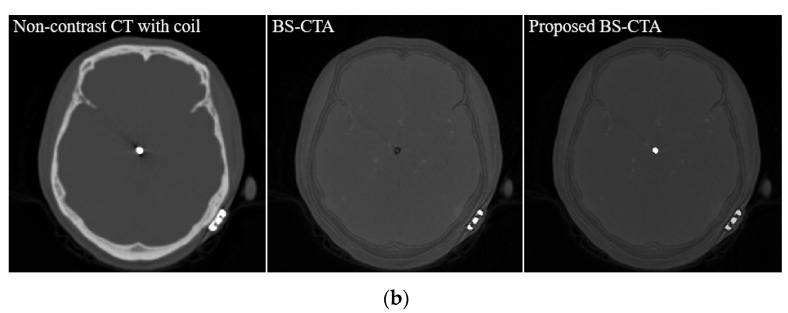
Results of non-contrast CT (left), BS-CTA (middle), and the proposed BS-CTA (right); (a) the two-clip case and (b) the coil case.
